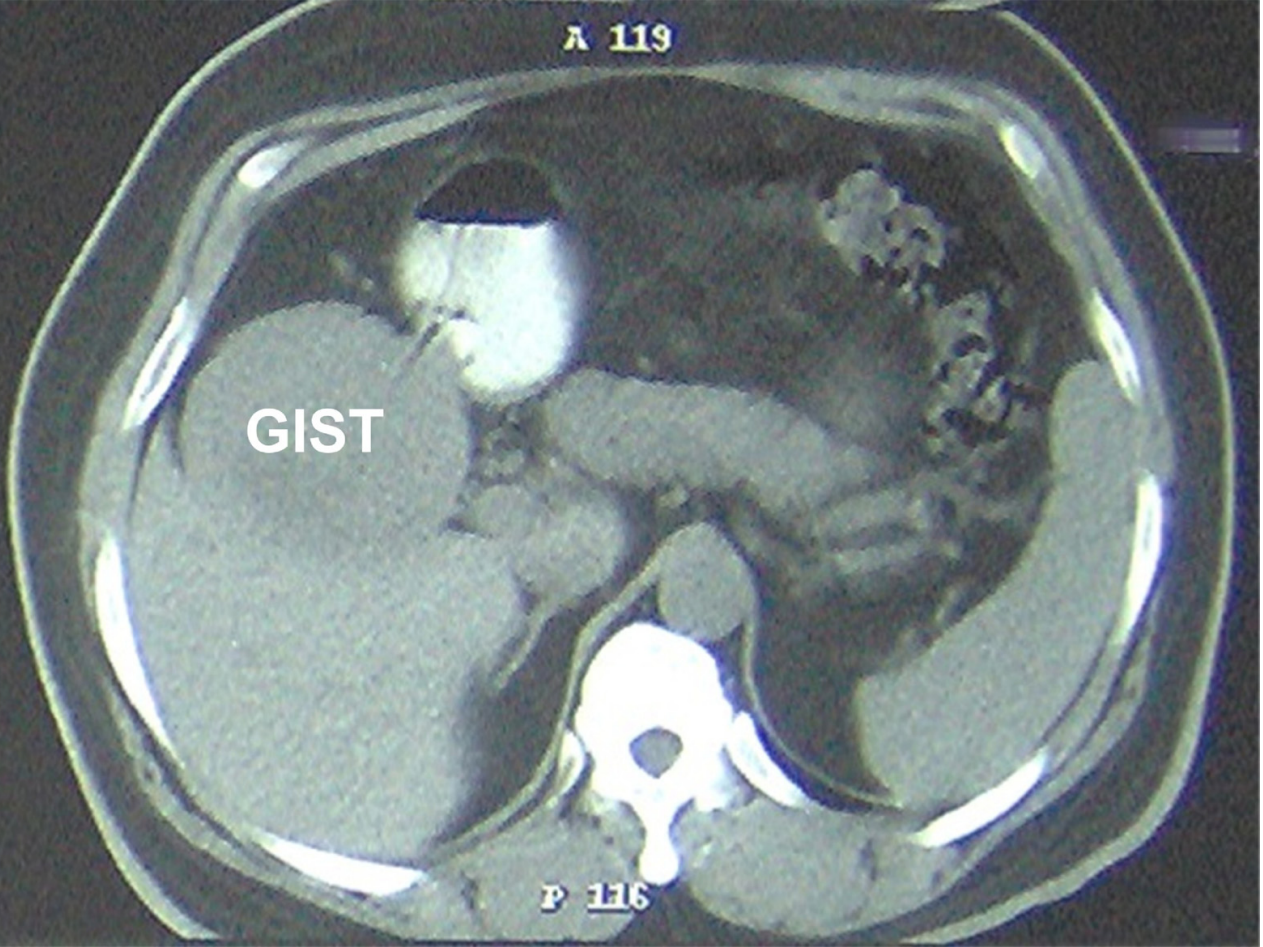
CT scan of patient showing duodenal GIST.
